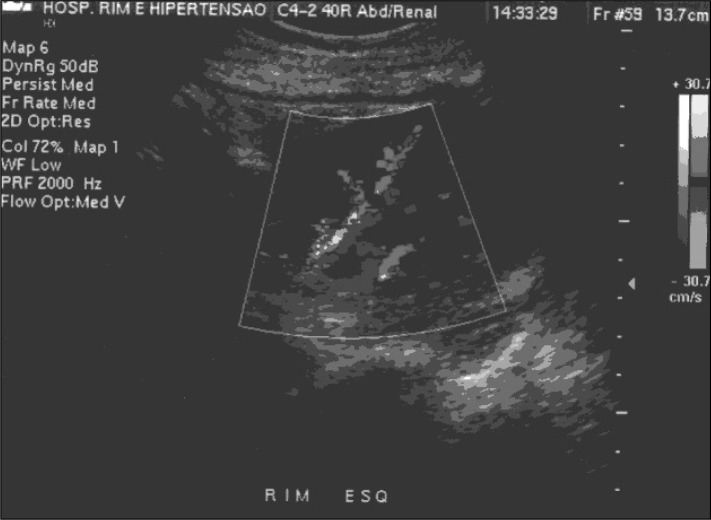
Left renal Doppler ultrasound : inferior cava thrombosis.
